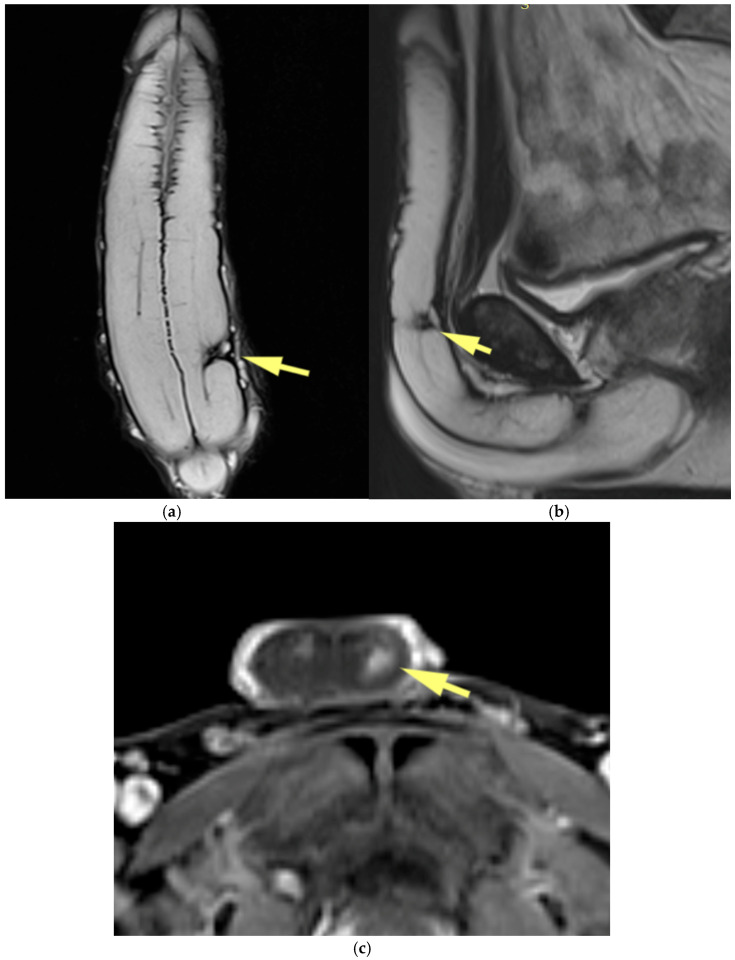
Peyronie’s disease. (a) Coronal and (b) sagittal T2-weighted images show a hypointense plaque (yellow arrow) in the mid-portion of the left corpus cavernosum. (c) Axial contrast-enhanced T1-weighted image demonstrates avid enhancement of the plaque (yellow arrow), indicating active disease.
